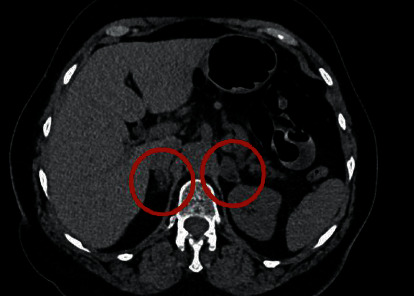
Follow up CT of the abdomen (horizontal plane).
